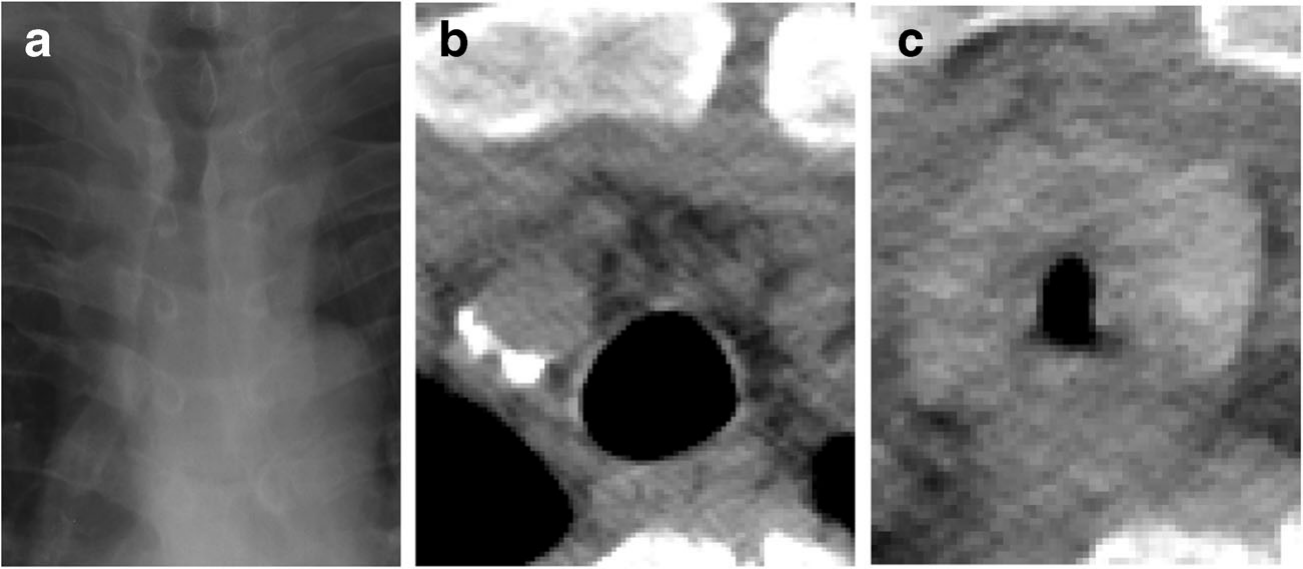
Fifty-two-year-old man with history of traumatic brain injury and extended intubation. a Chest radiograph for a syncopal episode demonstrating an hourglass shape of the thoracic trachea. b Pre- and c Post-intubation axial CTs of the same patient demonstrating interval focal narrowing of the trachea, likely iatrogenic
